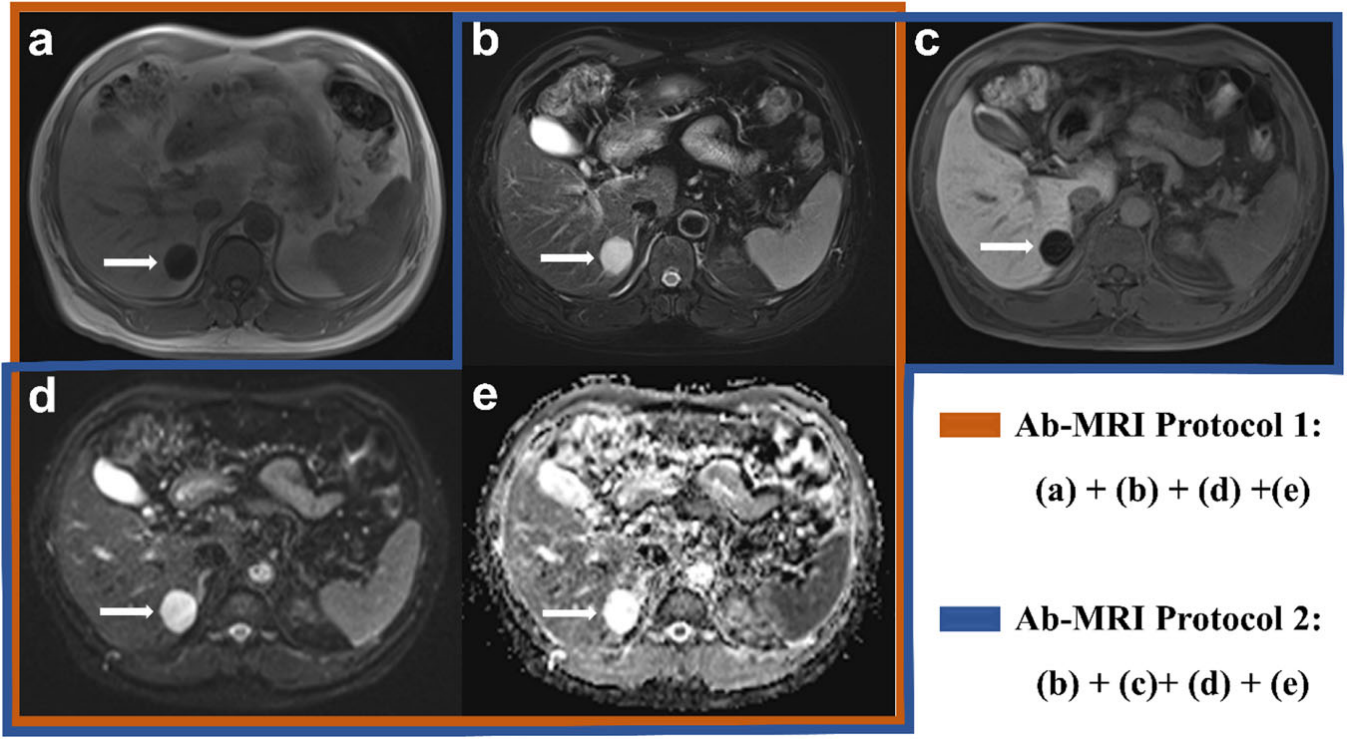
Display of two abbreviated MRI protocols in a 47-year-old male patient. a Dixon T1-weighted images (in-phase), (b) turbo spin-echo T2-weighted image, (c) hepatobiliary phase (HBP), (d) high b-value diffusion-weighted image (DWI), and (e) apparent diffusion coefficient (ADC) map. In segment VI, a lesion (arrow) was visualized that was characterized by low signal intensity on T1-weighted imaging, contrasting with elevated signal intensities on both DWI and T2-weighted sequences, coupled with a markedly high ADC value. Notably, this lesion showed marked hypointensity on HBP. Consistently, the lesion was assigned a consensus grade of 1 by two independent readers using two abbreviated MRI protocols, correlating with a diagnosis of hepatic cyst
